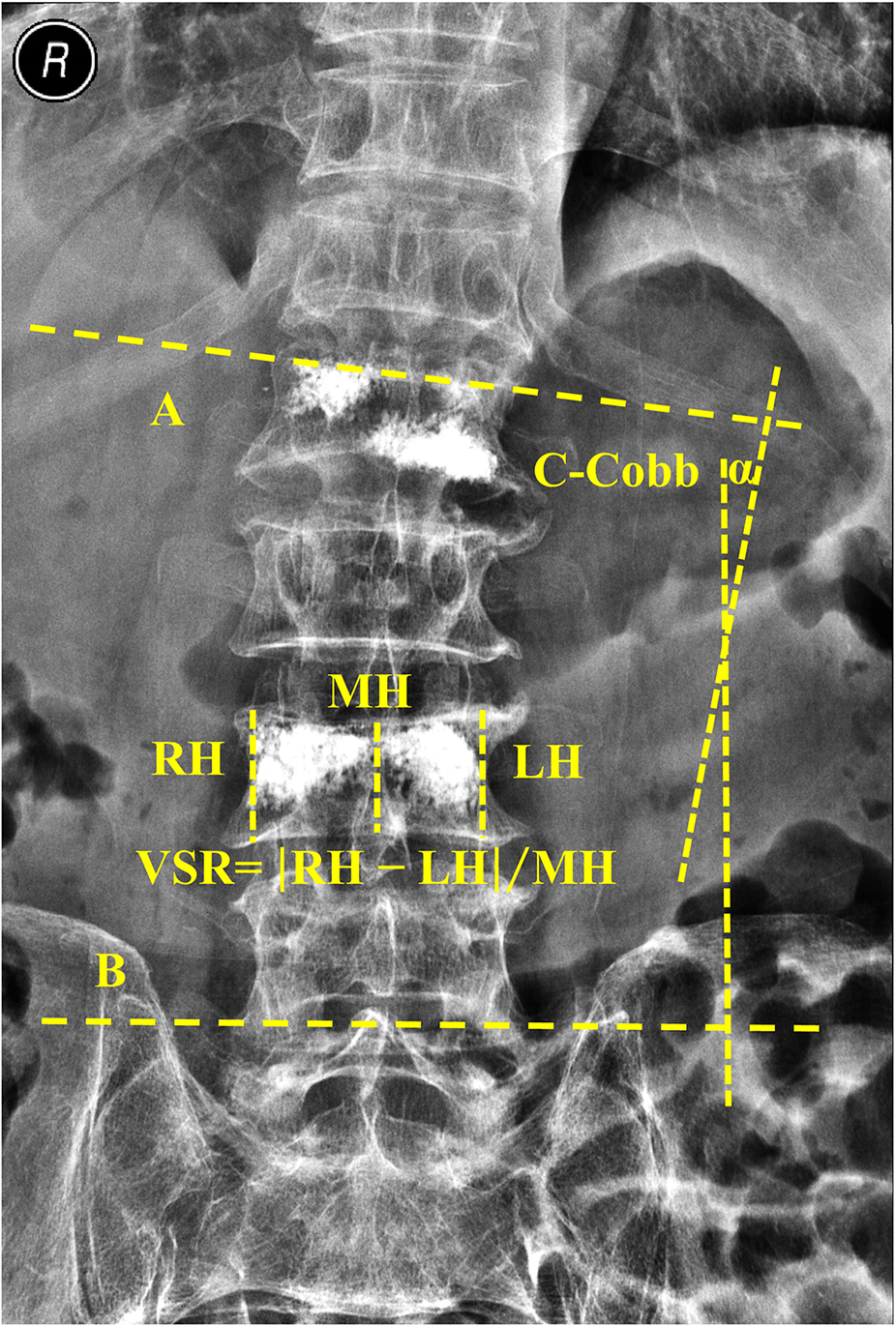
Measurements of coronal segmental cobb (C-cobb) and the vertebral side ratio (VSR). The heights of both left (LH), right (RH), and middle (MH) vertebral bodies were measured through an anteroposterior radiograph as shown in the Figure. The VSR was calculated as the following formula: VSR = |RH–LH|/MH. The Coronal Segmental Cobb (C-Cobb) angle α was measured from an anteroposterior radiograph by recording the angles between line A and line B, which were drawn parallel to the cranial and caudal most-tilted vertebral endplates.
